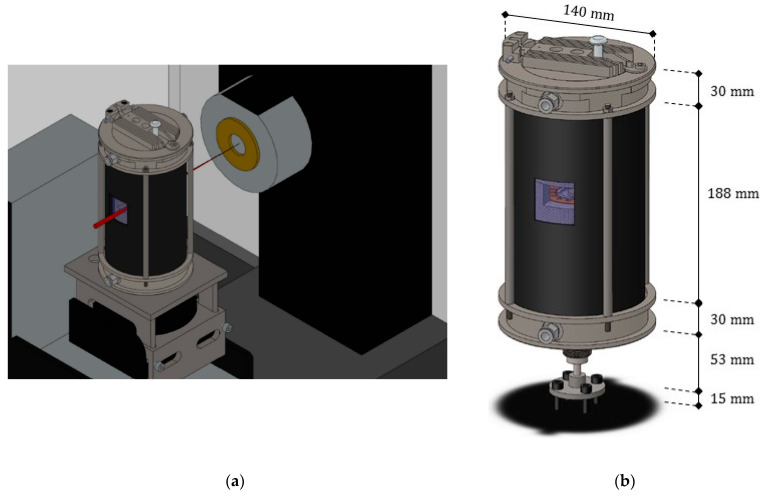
(a) Axonometric view of the furnace positioned within the cabinet, with a pictorial representation of the X-ray beam (colored in red) emitted by the tube; (b) enlarged view of the prototype, with overall dimensions.
